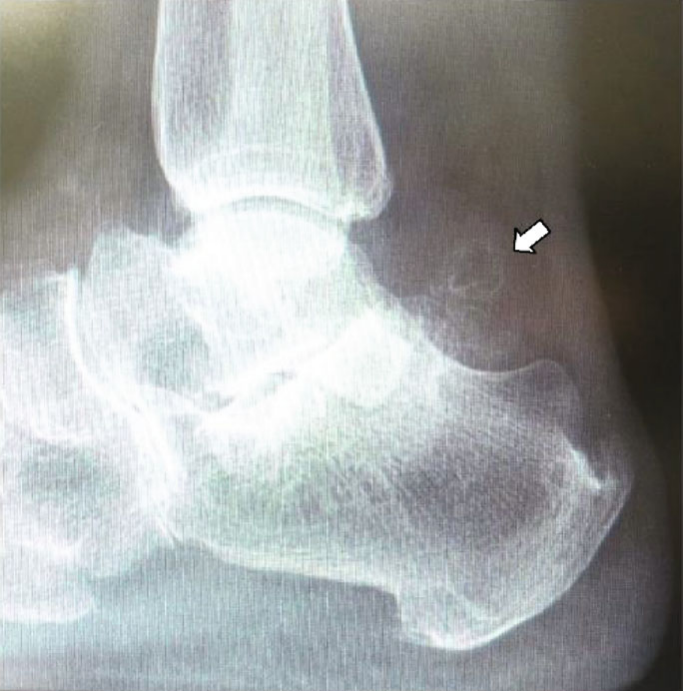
Lateral plain radiographic view of the right ankle obtained preoperatively showing several ossified masses in the posterior ankle area (arrow).
